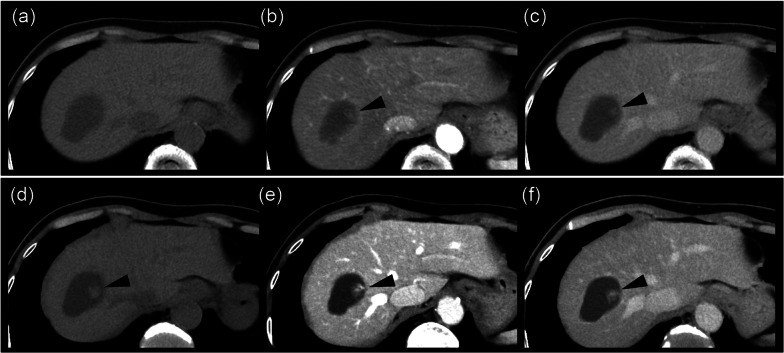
Non-contrast (a, d), arterial phase (b, d), and portal venous phase (c, e) computed tomography (CT) images obtained one year before surgery (a–c) and immediately before surgery (d–f). Over approximately one year, the irregularly shaped mural nodule (arrowheads) within the cystic lesion showed an increase in size and the degree of contrast enhancement
